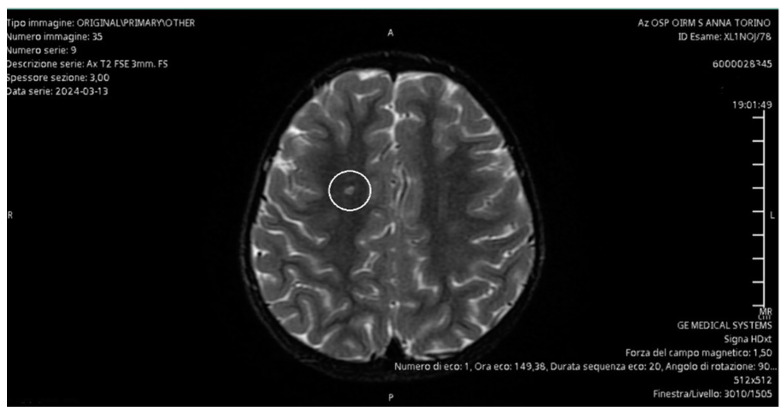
Magnetic resonance imaging scan shows a small vascular lesion on a probable microembolic basis at the right semioval center.
